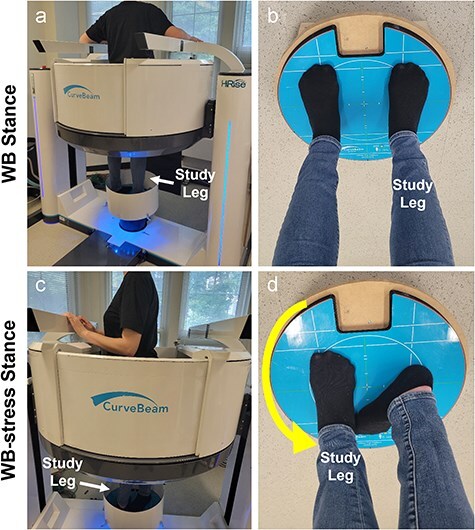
(a) Participant standing in the Curvebeam High Rise® weight-bearing CT scanner for the neutral WBCT scan of the hip. (b) Demonstration of the foot position on the foot platform for the neutral (WB) scan. (c) Patient inside the WBCT scanner demonstrating patient-selected maximal external rotation during the stress (WB-stress) scan. (d) The foot on the study leg was shifted to the foot location of the contralateral leg during the neutral scan, and the patient was instructed to rotate their body on the study leg to bring their opposite shoulder as far backward as possible. For this case, the right hip is the study hip, so the left shoulder was rotated back in the scanner (toward the camera/viewer). The participant then brought their contralateral foot back onto the platform behind the foot of the study limb (d) and equalized weight for stability during scanning.
